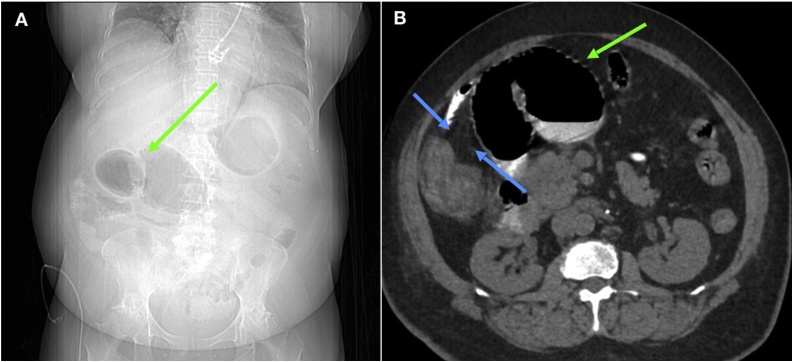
A. Scout film of abdomen taken prior to CT imaging upon admission in 2018 showing dilated loops of small bowel (green arrow). B. CT Abdomen and Pelvis upon admission demonstrating an obstructed and dilated loop of jejunum (green arrow). Multiple parallel fine linear densities consistent with Ladd bands (blue arrows) are seen extending from ascending colon and cecum.
